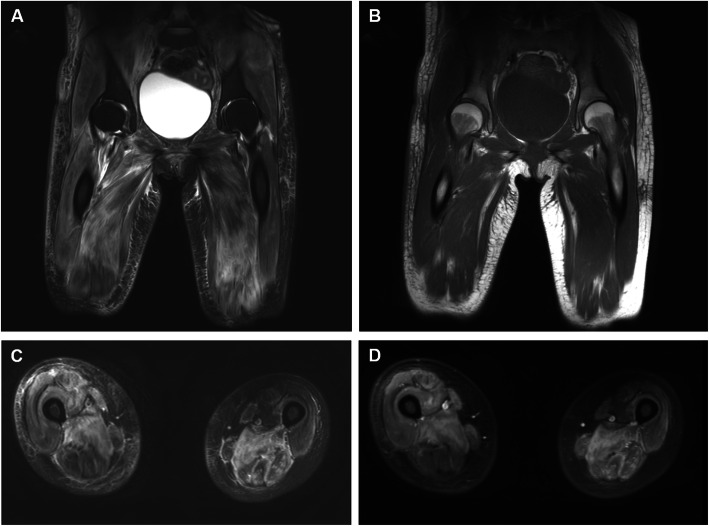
Magnetic resonance imaging of the thigh. Coronal images show A increased T2 signal intensity and B normal T1 signal intensity in the pelvis and proximal thigh. C Axial T2-weighted and D gadolinium-enhanced T1-weighted image shows symmetric diffuse muscle edema and inflammation with relative preservation in the left anterior compartment of the thigh
